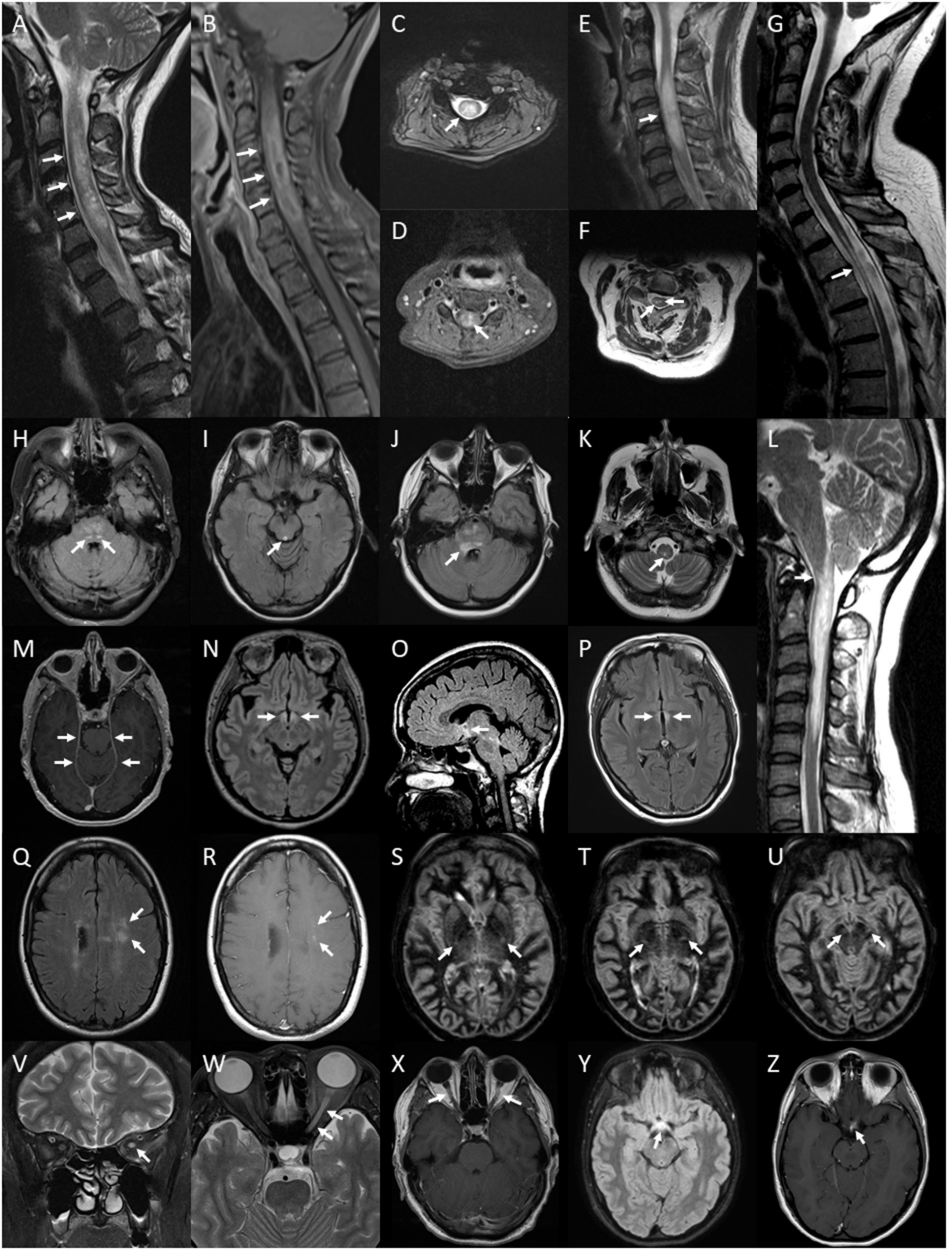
Lesions of the spinal cord, brain and optic nerve associated with or exclusively seen in NMOSD. Spinal cord lesions: longitudinally extensive spinal cord lesion (arrows) seen on T2 sagittal image of the cervical cord (A), peripheral Gd-enhancing lesion (arrows) seen on T1 sagittal image of the same lesion (B), central cord lesion (arrow) on T2 axial image at the level of C4 from the same lesion (C) and central Gd-enhancement (arrow) on T1 axial image in the same region (D); swelling (arrow) of a high signal lesion on T2 sagittal image of the cervical region (E); bright spotty cord lesions (arrows) on axialT2 image of the cervical region (F); spinal cord atrophy with myelomalacia (arrow) on sagittal T2 image of the cervico-thoracic region (G); Brainstem lesions: bilateral nucleus tractus solitarius high signal lesions (arrows) on axial FLAIR image through the pons (H); periaqueductal high signal lesion (arrow) on axial FLAIR image through the midbrain (I); high signal FLAIR lesion involving the floor of the fourth ventricle on axial FLAIR imaging at the level of the pons (J); central medullary lesion (arrow) on T2 axial image of the medulla (K) and sagittal T2 image of high cervical cord lesion showing extension into the medulla (L). Leptomeningeal enhancement of the tent (arrows) on axial gadolinium enhanced T1 image at the level of the midbrain (M). Brain FLAIR lesions: hypothalamic high signal lesion (arrows) on axial image (N) and midline sagittal image (O); high signal lesion involving the walls of the third ventricle (arrows) on axial image (P). Cloud-like Gd-enhancing lesions (arrows) shown on axial FLAIR image (Q) and Gd-enhanced T1 image (R). Bilateral longitudinally extensive cortico-spinal tract lesions (arrows) seen on sequential axial DIR images through the basal ganglia and midbrain (S–U). Optic nerve lesions: high signal lesion of the left optic nerve (arrow) on coronal T2 image of the orbits (V); longitudinally extensive high signal lesion of the left optic nerve (arrows) on axial T2 image of the orbits (W); bilaterally Gd-enhancing lesions of the optic nerves (arrows) on axial Gd-enhanced T1 image of the orbits (X); optic chiasm lesion (arrow) on axial Flair image (Y) and Gd-enhanced T1 image (Z).
